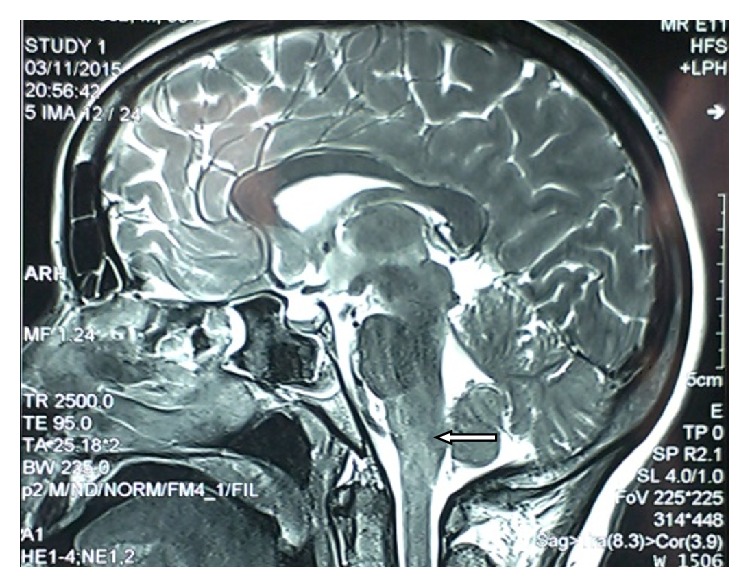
MRI of the brain in sagittal section showing hyperintensities at the cervicomedullary junction (white arrow).
